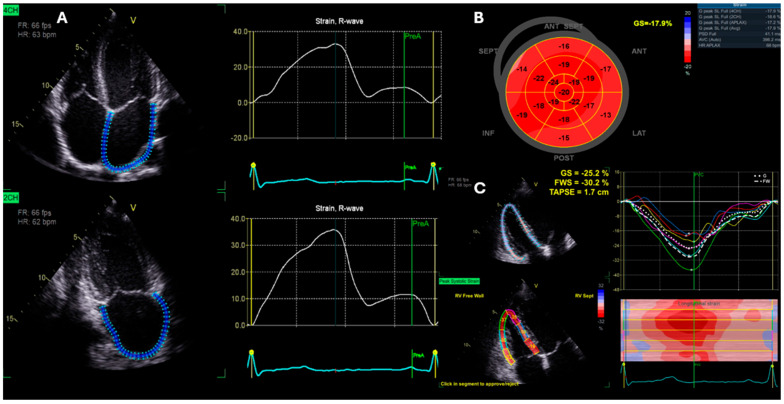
Example of Speckle tracking echocardiography for the assessment of left atrial reservoir, conduit and booster strain (A), global longitudinal left ventricular strain bullseye (B) and right ventricular free wall strain (C).
